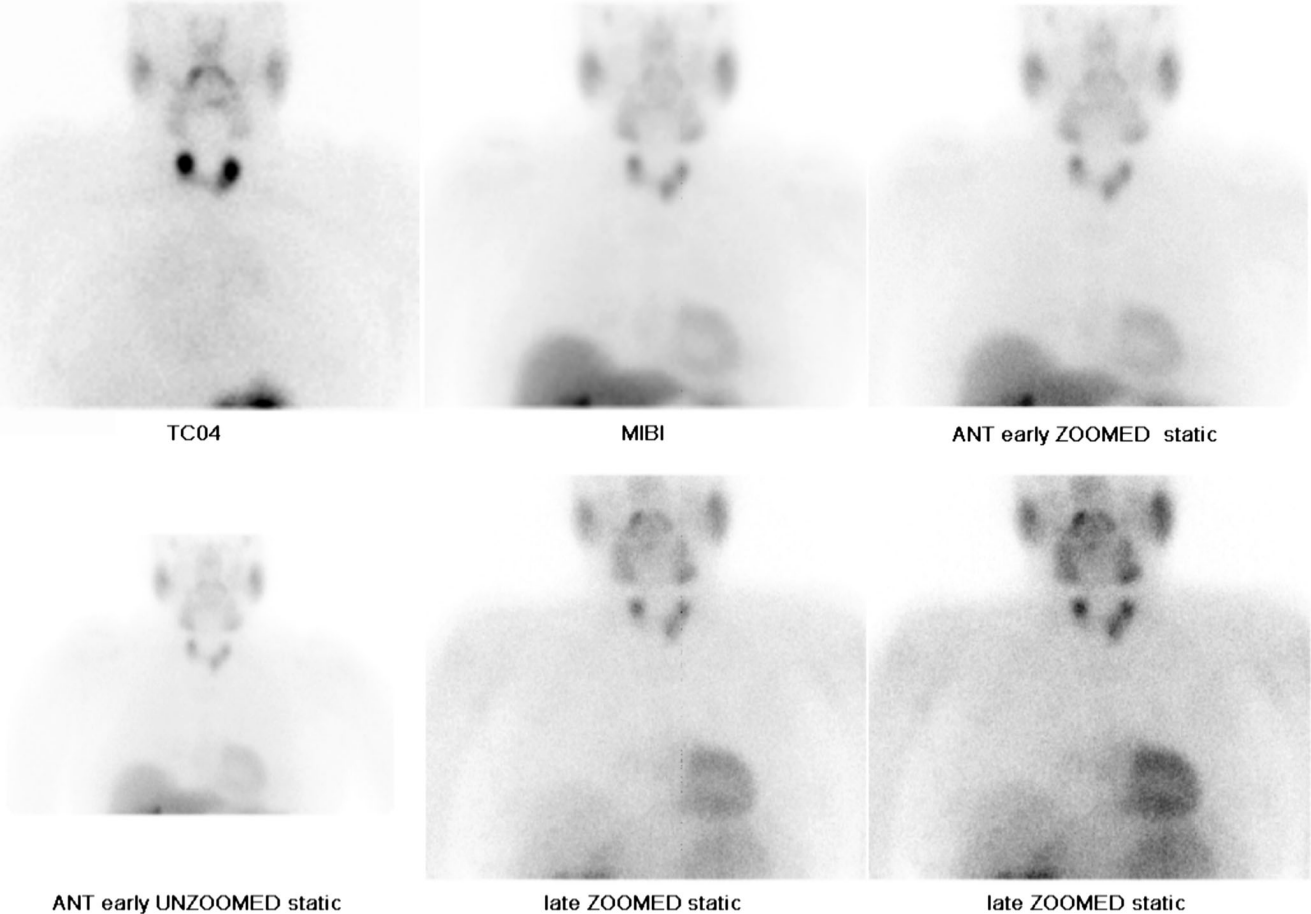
A nuclear medicine parathyroid scintigraphy study (image courtesy of Dr Cindy Leung, Barts and The London). The Technetium pertechnetate (^99m^Tc-pertechnetate) image (upper row, far left) demonstrates localization of the thyroid gland. ^99m^Tc-Sestamibi (MIBI) images show a focal area of significant increased tracer uptake inferior to the left thyroid lobe, which persists on early (centre and far right, upper row) and delayed imaging (centre and far right, lower row). Imaging characteristics are consistent with a parathyroid adenoma. Tracer uptake elsewhere is physiologicalHyperparathyroidism and hypercalcaemia are often more profound in parathyroid carcinoma compared to parathyroid adenomas; however, the imaging appearances can be indistinguishable although parathyroid carcinomas are typically larger and may demonstrate invasive features, e.g., invasion of the prevertebral muscles and surrounding structures (Fig. 11) [14]. Therefore, in the absence of obvious metastatic disease, the diagnosis is typically confirmed following surgical resection.
